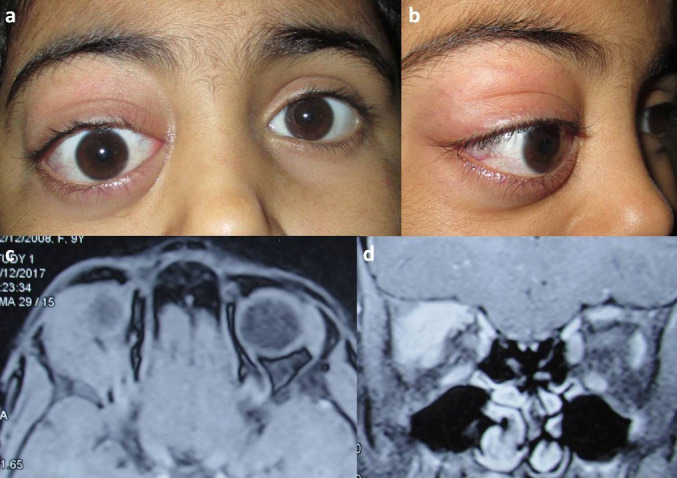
a Primary gaze - OD shows inferior dystopia with conjunctival congestion; b. The lateral view shows supero-temporal sulcus fullness, proptosis, and conjunctival congestion c. MRI (axial, T2W, superior) shows a hyperintense soft-tissue lesion in supero-temporal orbit (lacrimal gland region) with extension into posterior orbit; d. MRI (coronal, posterior) soft-tissue lesion in the extraconal compartment with an infero-medial displacement of orbital contents i.e., extraocular muscles
